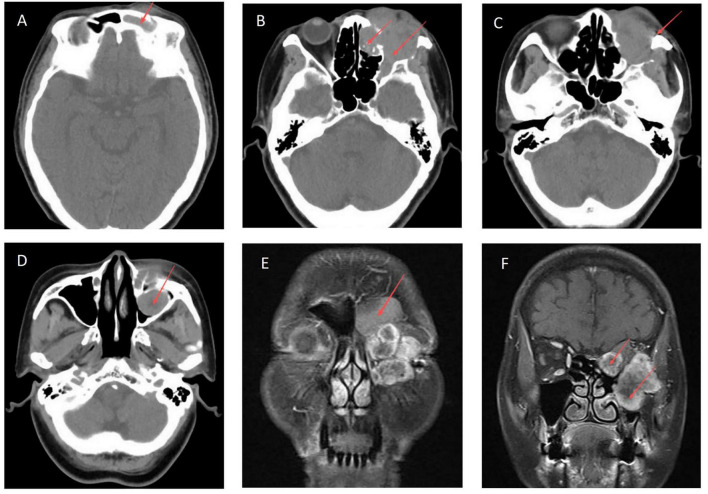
Computed tomography (CT) and MRI (T1) revealed an irregular soft tissue mass was observed within the left orbit, extending medially into the frontal (A,E) and ethmoid sinuses (B,F), laterally to the zygomatic arch (C), and inferiorly into the maxillary sinus (D,F), with obliteration of the intraorbital fat planes (B).
